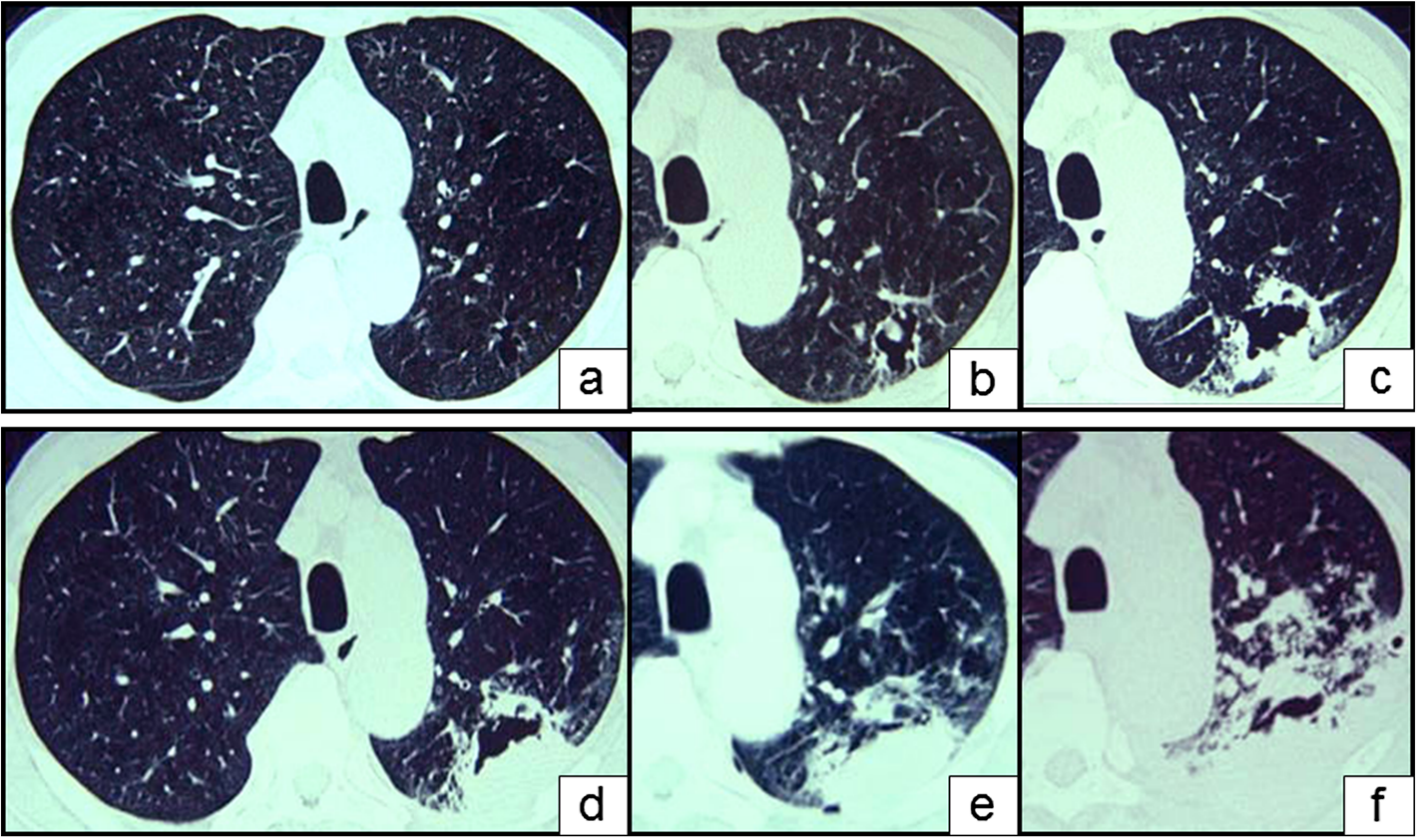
Computed tomographic imaging of the chest at the level of the aortic arch in 2003, two years before dyspnea onset, showed a small cavity in the left upper lobe due to emphysematous change (a). Computed tomography 2 years after initial presentation (April 2005) showed that the cavity was increasing in size and contained a fungus ball (b). The cavity then continued to increase in size, developing a thick wall and infiltration of the surrounding areas, with increased pulmonary effusion (c: July 2005; d: October 2005; e: December 2005; f: January 2006).
